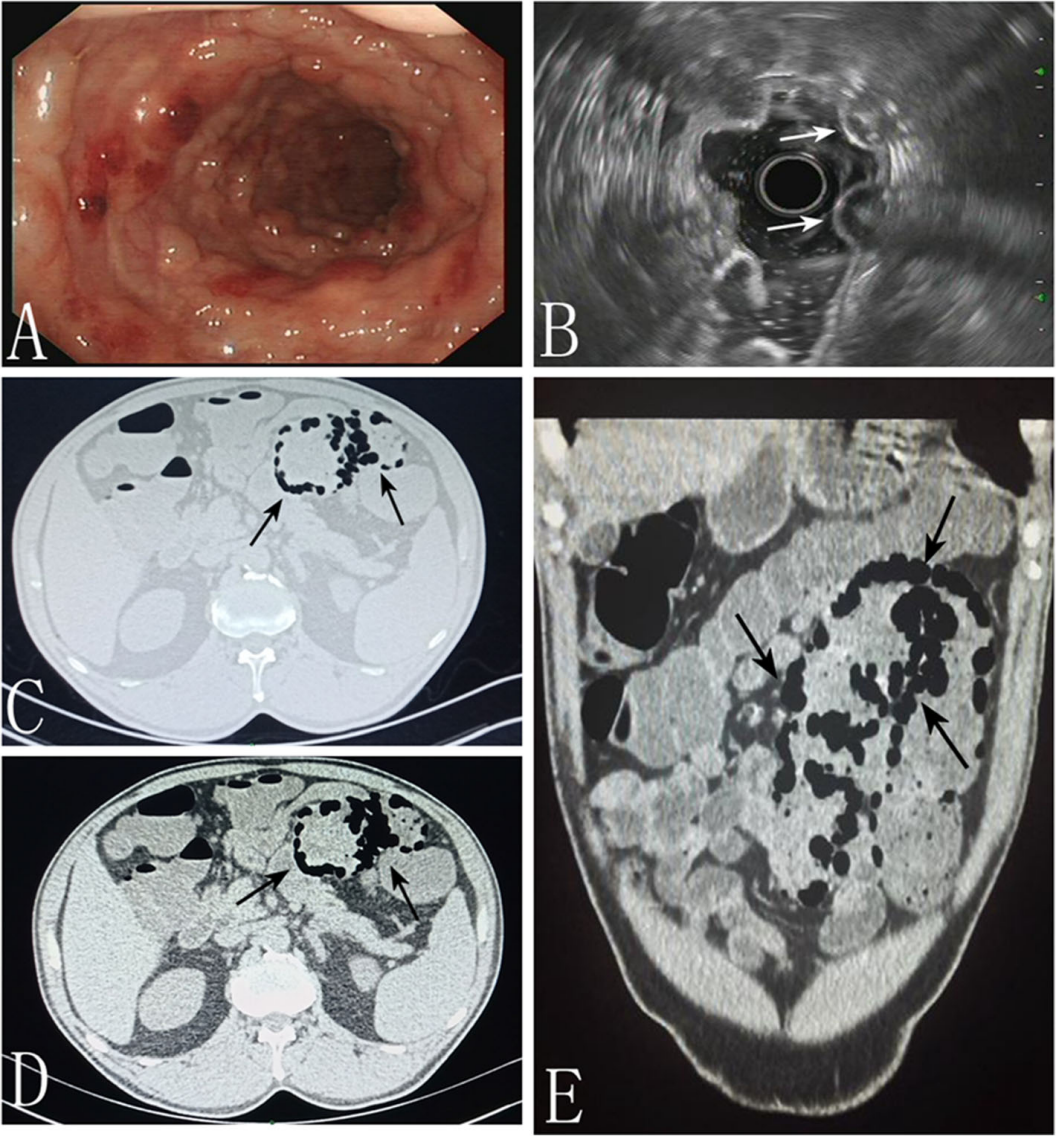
Endoscopic and CT examination. a Colonoscopy disclosed multiple nodular lesions in the transverse colon and sigmoid colon. b Endoscopic ultrasonography showed hypoechoic lesions (white allows). c CT Horizontal scan. Image adjusted to lung window (black allows). d CT Horizontal scan. Image adjusted to abdominal window (black allows). e CT Vertical scan. Multiple grape-like gases were visible in sigmoid colon ((black allows)
